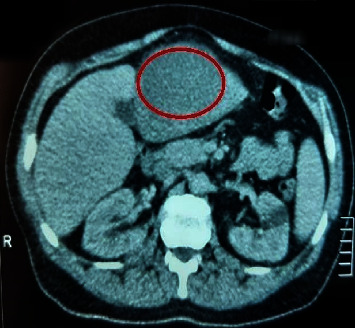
Axial contrast-enhanced CT demonstrates a large cystic lesion with a relatively thin wall in the hepatic segment IVb. CT findings are compatible with hepatic abscess.
